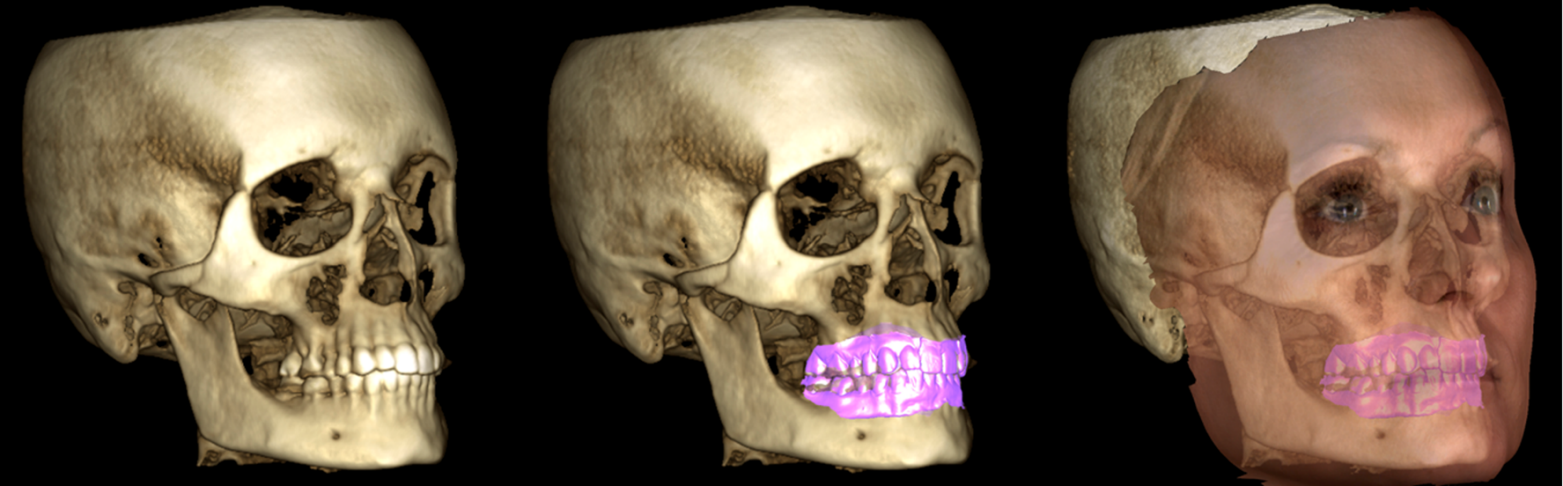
CBCT examination combined with 3D photography. (Courtesy of the manufacturer. The image is shown with the patient's permission)
